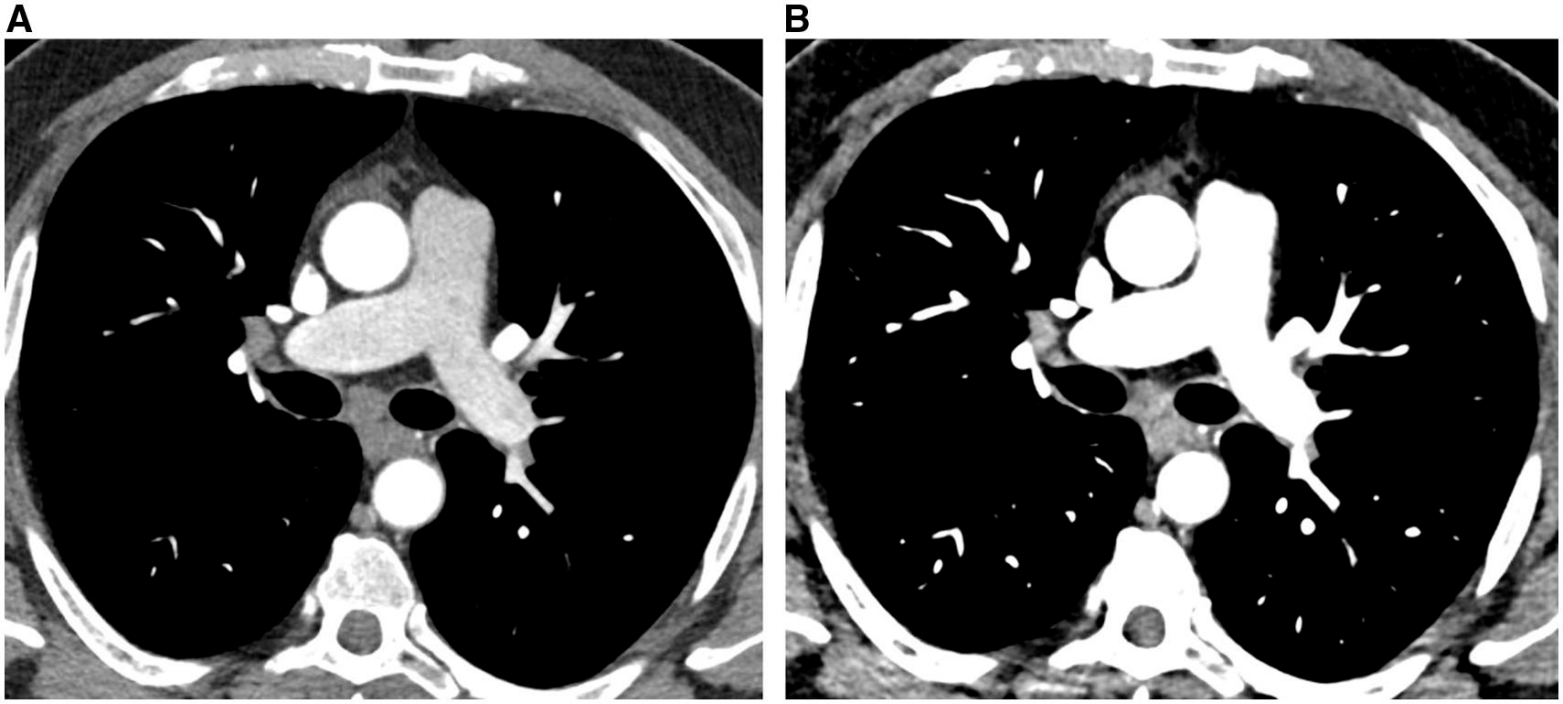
Example of a 45-year-old male patient referred for suspicion of acute pulmonary embolism. Chest CT angiographic examination obtained with PCD-CT (0.4 mm collimation; pitch of 1). (A) Axial PCD-CT mediastinal image reconstructed at 70 keV, showing suboptimal opacification of pulmonary arteries secondary to a Valsalva manoeuver. (B) Axial PCD-CT mediastinal image reconstructed at 40 keV. The low-energy reconstruction enables restoration of optimal enhancement of pulmonary arteries. The quality of vascular opacification enabled confident exclusion of acute pulmonary embolism down to the subsegmental level.
